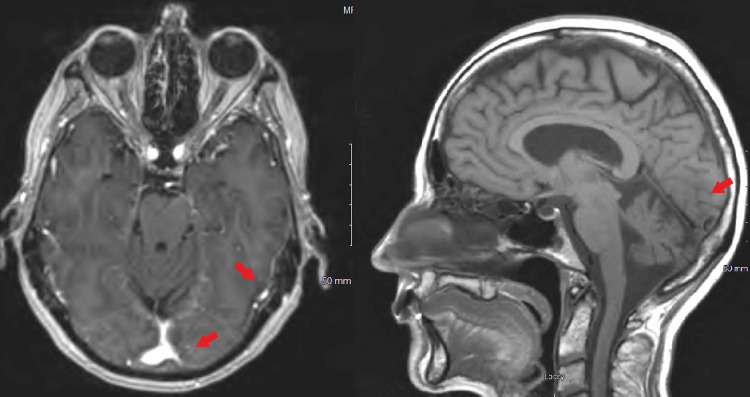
MRI of the brain with and without contrast These images demonstrate an acute infarct in the left occipital lobe (arrows). Additional small punctate areas of acute infarction are present in the left parietal deep white matter and left thalamus, which are findings suggestive of an embolic phenomenon. A small enhancing focus is also noted in the right parietal region, which may represent a prominent dural vessel; however, a small focus of dural metastasis cannot be excluded. MRI: magnetic resonance imaging
